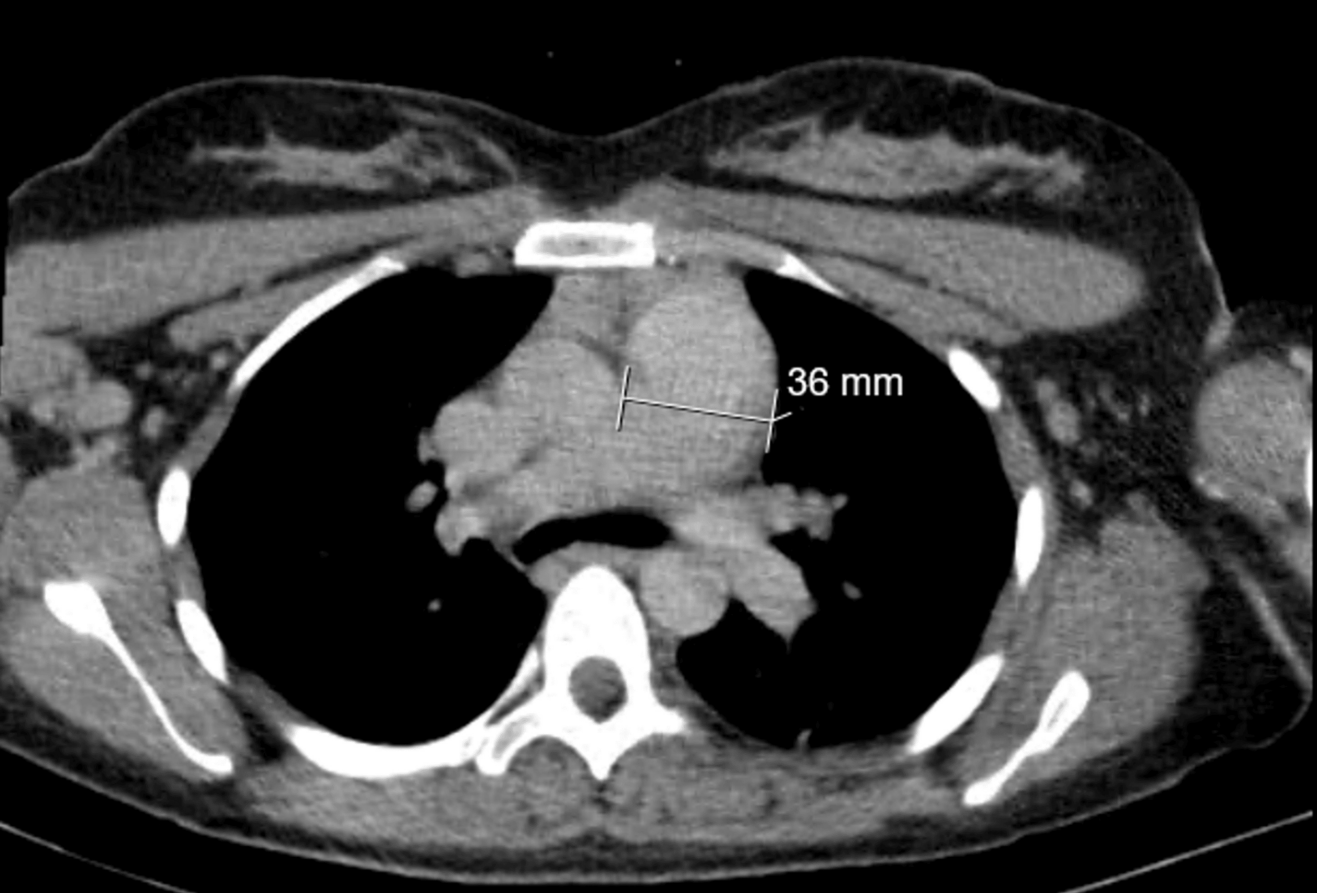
Thoracic CT in the axial plane obtained prior to admission to the intensive care unit
